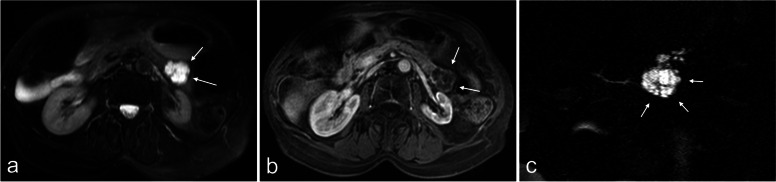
Pancreatic MRI performed 13 years earlier showed a typical pancreatic microcystic serous cystadenoma in the tail of pancreas. a Axial T2-weighted image. Well-defined, homogeneous, high T2 signal intensity, polycystic components (arrows). b Axial T1-weighted image after gadolinium chelates (Dotarem®) injection. Isolated septa enhancement (arrows). c Radial MR cholangiopancreatography. Honeycomb pattern (arrows)
